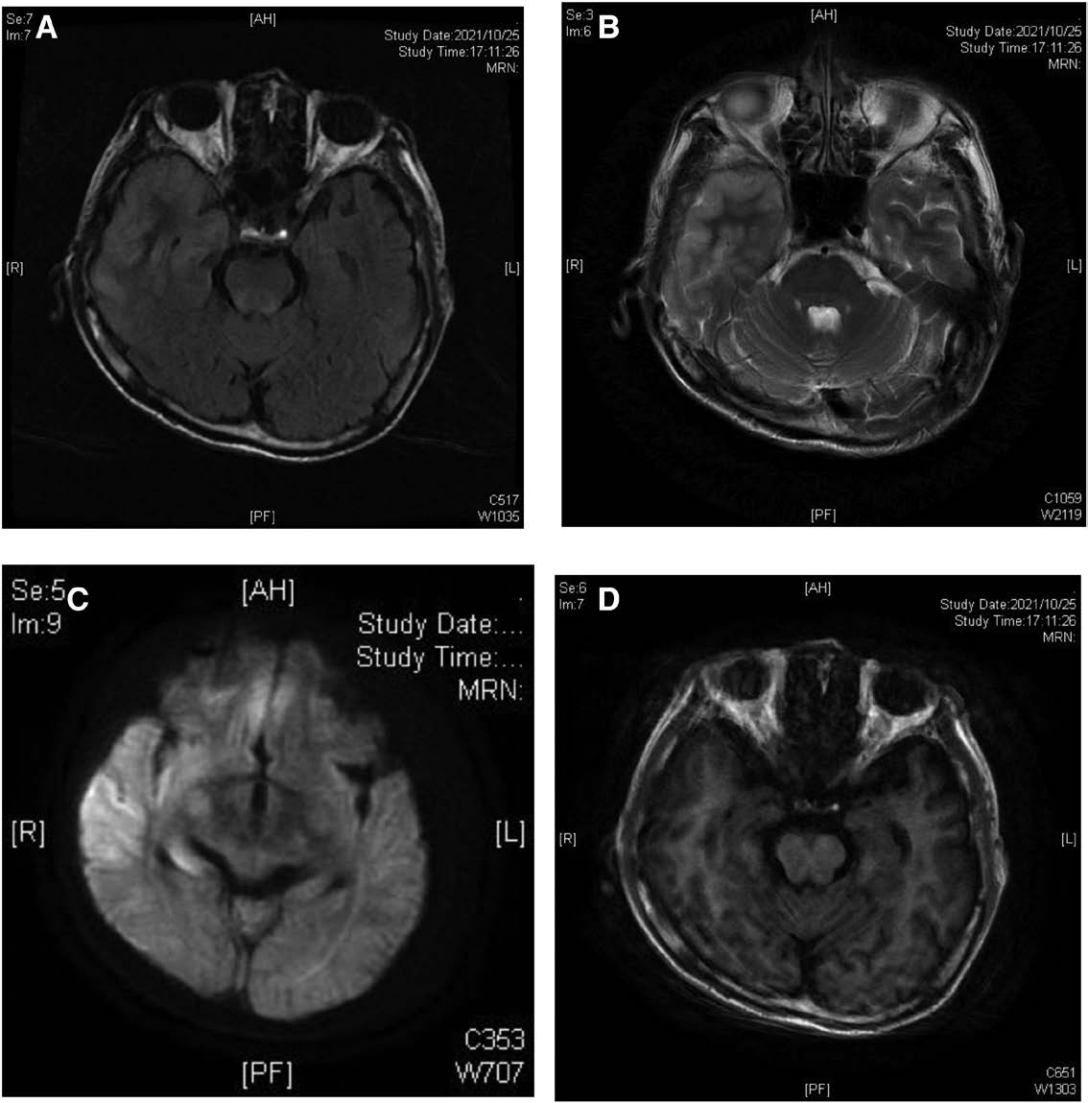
(A) T2 image, slightly hyperintense patchy lesion in the right temporal lobe. (B) FLAIR image, slightly hyperintense signal in the right temporal lobe hippocampus. (C) DWI shows high signal in the temporal and insular lobes. (D) No significant changes observed in the T1 image.
